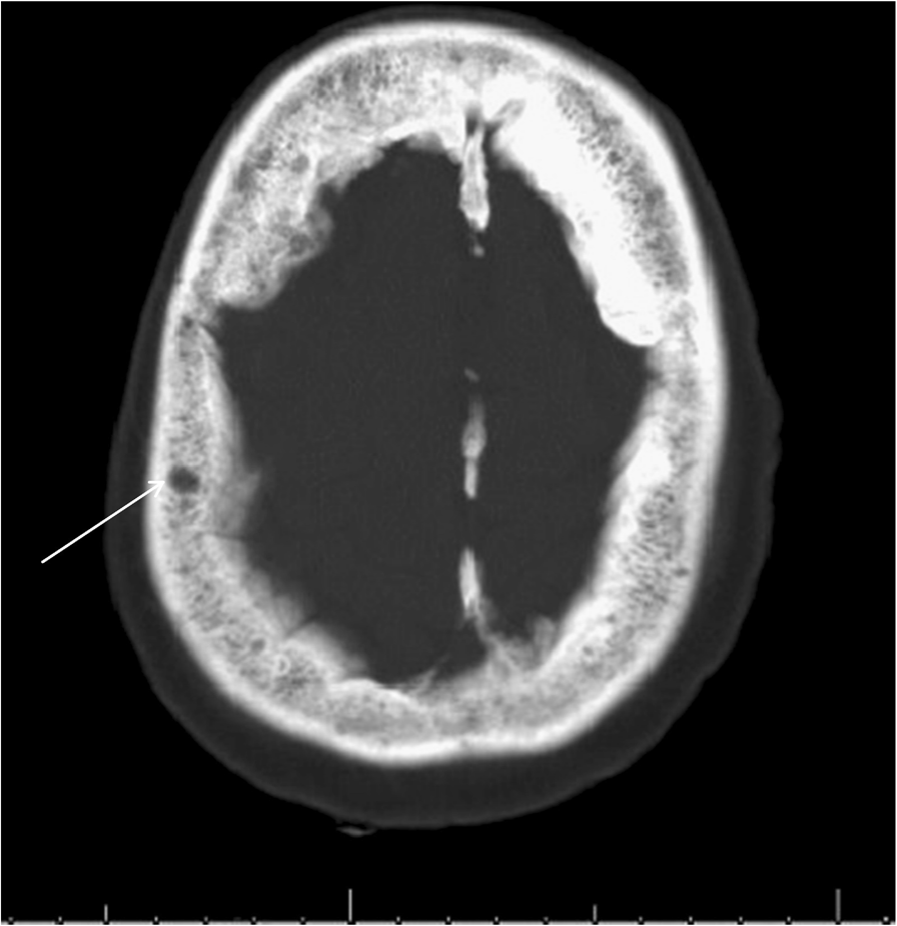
Computed tomography of brain (bone window). Computed tomography brain scan showing multiple lytic lesions in the calvarium (arrow).
